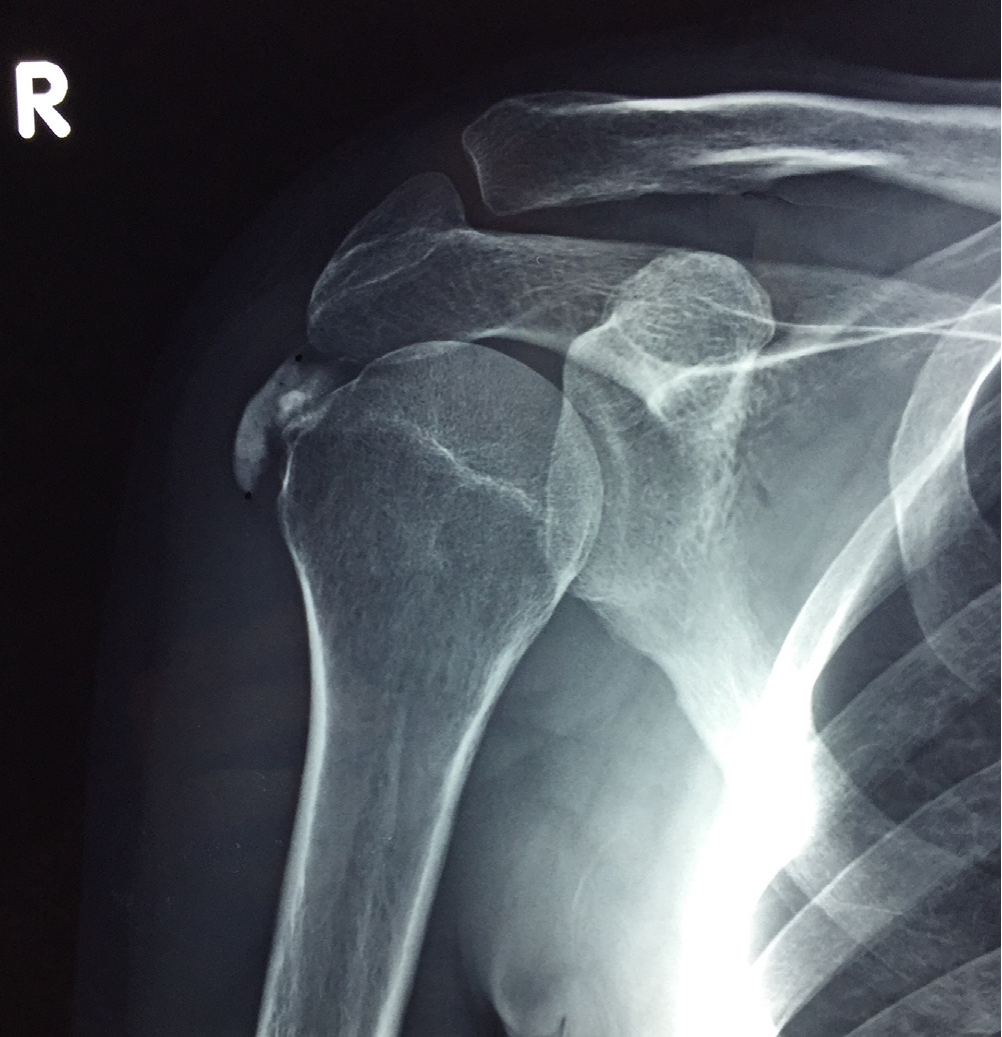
X-ray of a 33-year-old female patient who works as a receptionist. A calcific deposit was found in the insertion of the supraspinatus tendon.
